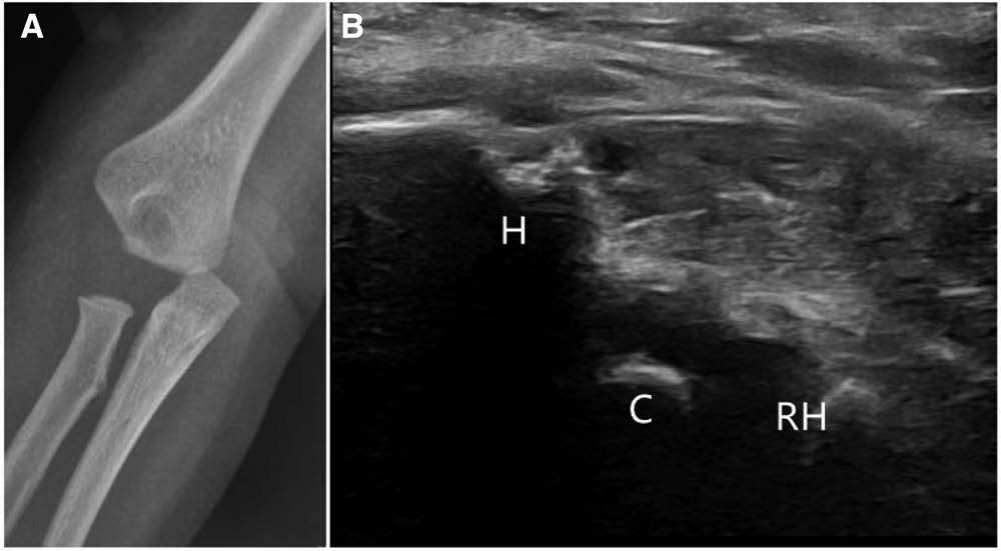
A 1-yr-old boy was diagnosed with a transphyseal separation of the distal humerus. (A) X-ray showed a dislocation-like fracture of the elbow joint. (B) The coronal longitudinal scan of ultrasound showed that there was a normal alignment in radio-capitellum joint and the capitellum was separated from distal humer. C = capitellum; H = humer, RH = radial head.
